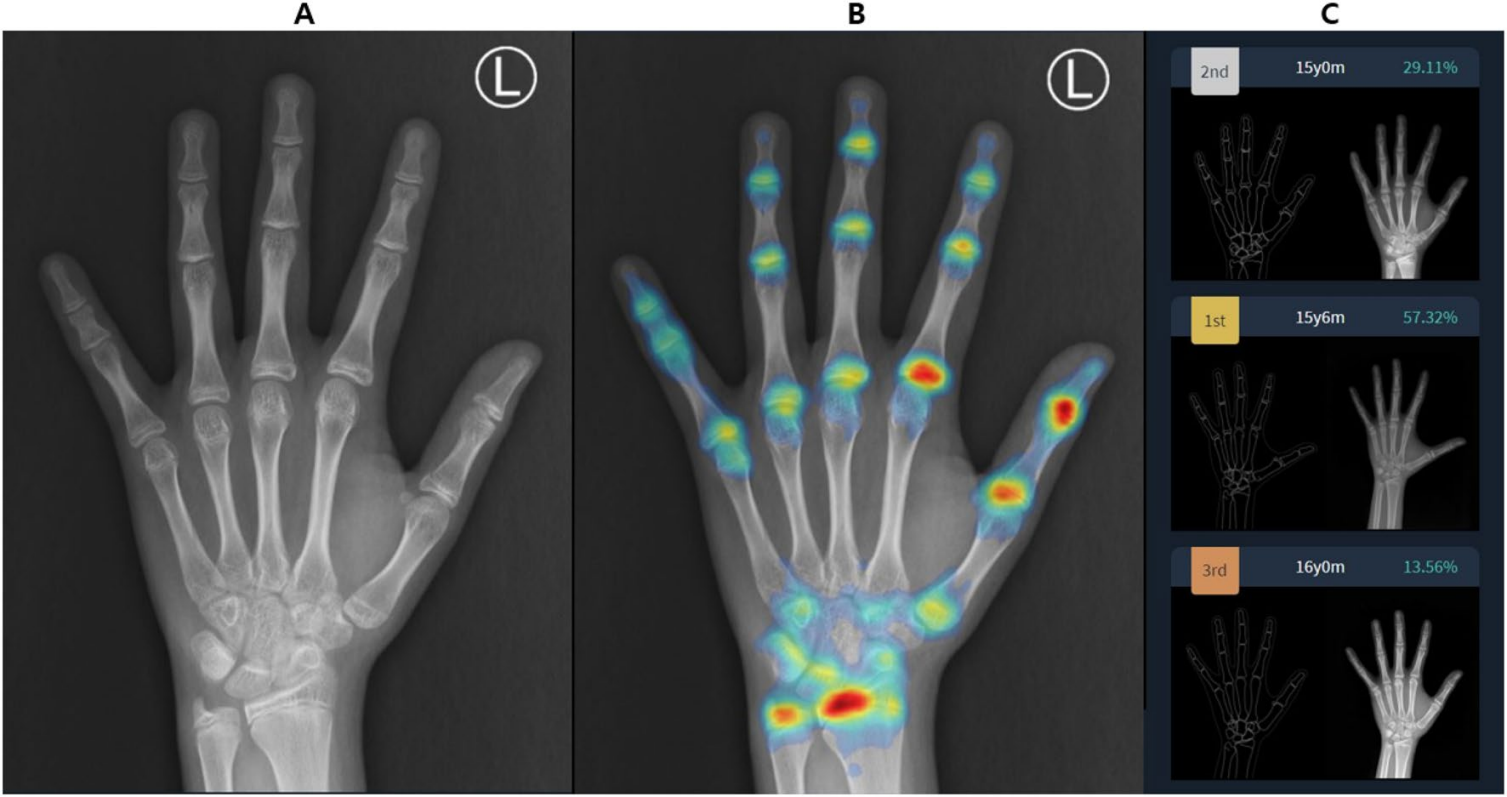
Screenshot of BA assessment by the software. Subject is a male patient with chronological age of 15 years and 3 month. (A) The software analyses the received left hand radiograph and (B) generates attention map using shape and density of each bone, then (C) displays estimated bone ages and each probability according to the attention map. First-rank bone age of this patient is 15 years and 6 months years with probability of 57.32%. The reference radiograph and outline-image depicting a specific shape of each bone are also shown in (C).
